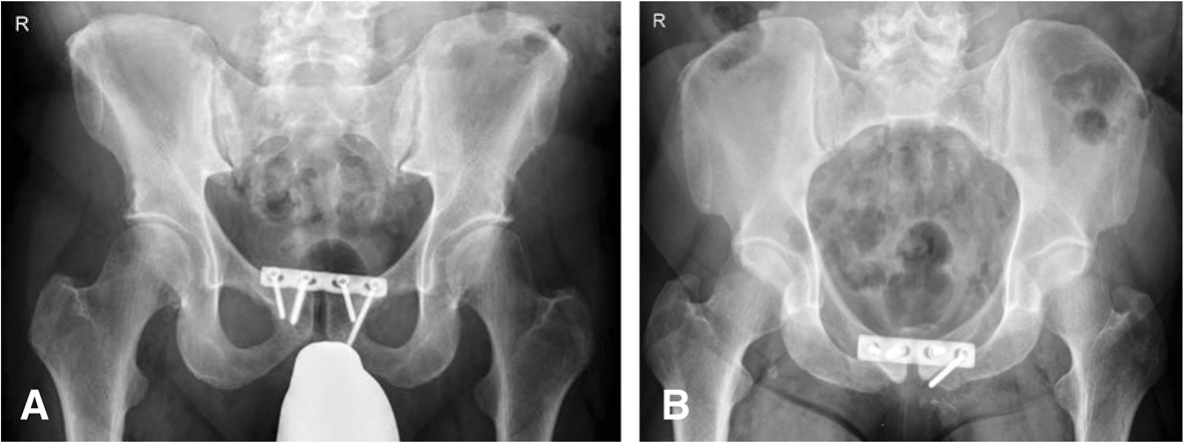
Postoperative plain pelvic radiographs. Anteroposterior (a) and inlet (b) view. Plating osteosynthesis of the pubic symphysis results in anatomical reduction and stabilization of the pelvic ring
